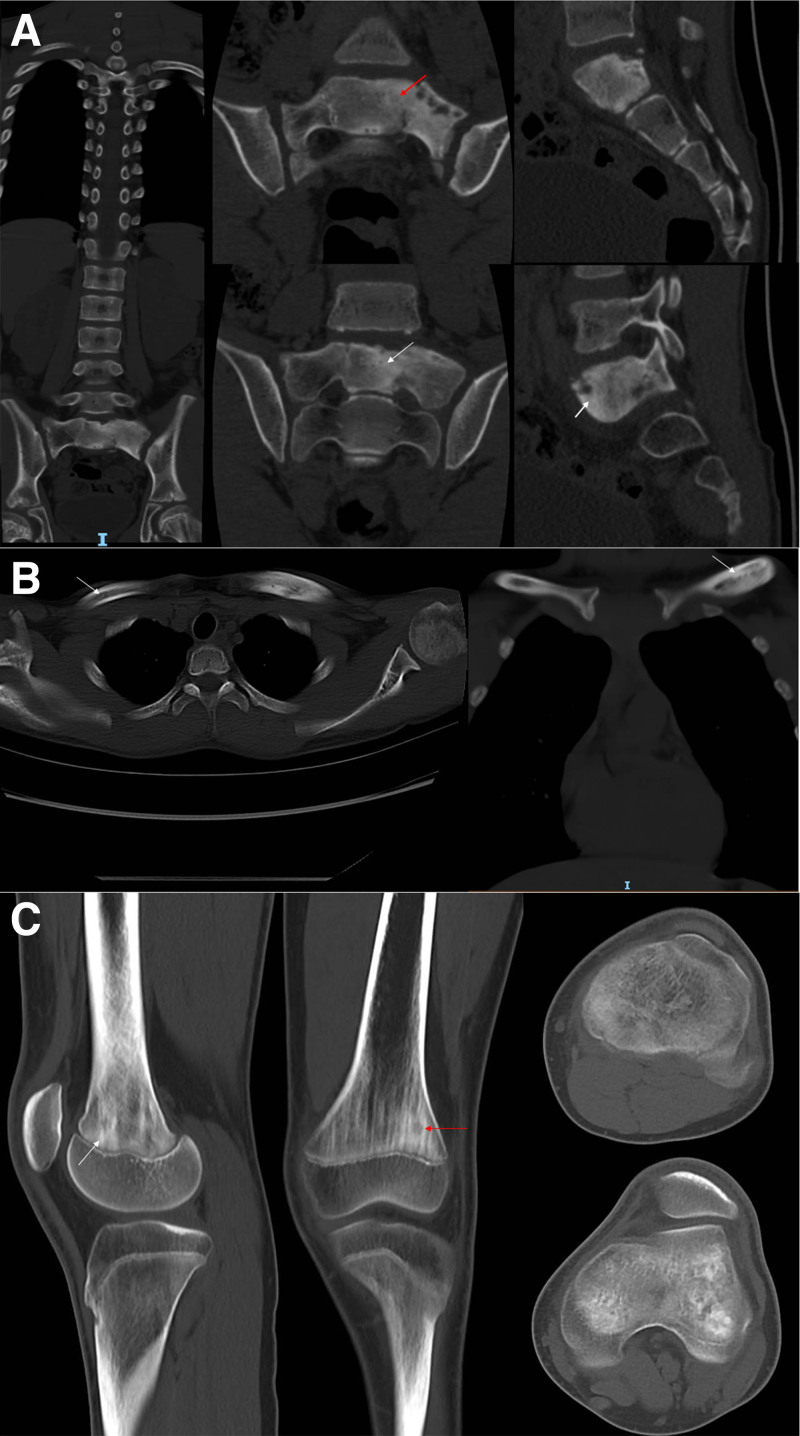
Findings of computed tomography in CRMO patients. (A) Lytic lesion (red arrow) and sclerosis (white arrow) of the sacrum in an 11-year-old boy; (B) intramedullary heterogeneous density (white arrow) of the clavicle in a 12-year-old girl; (C) lytic lesion (red arrow) and sclerosis (white arrow) of the sacrum in a 13-year-old boy. CRMO = chronic recurrent multifocal osteomyelitis.
